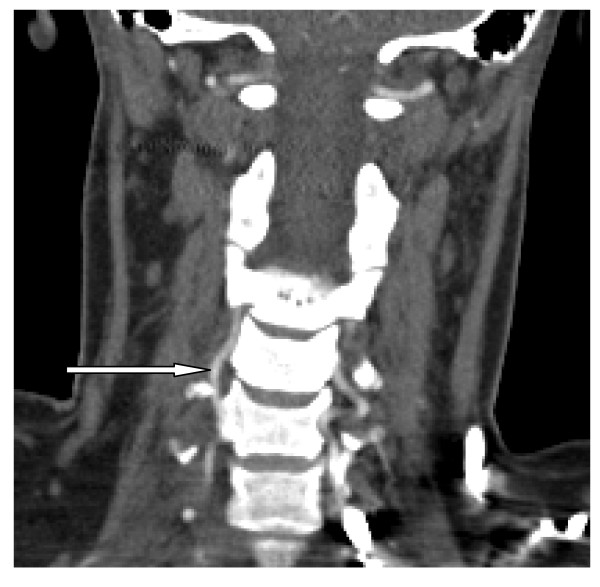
CTA done three months later using a 64-slice coronal reconstruction. There is normal blood flow at the level of C4 to C2 (white arrow).
